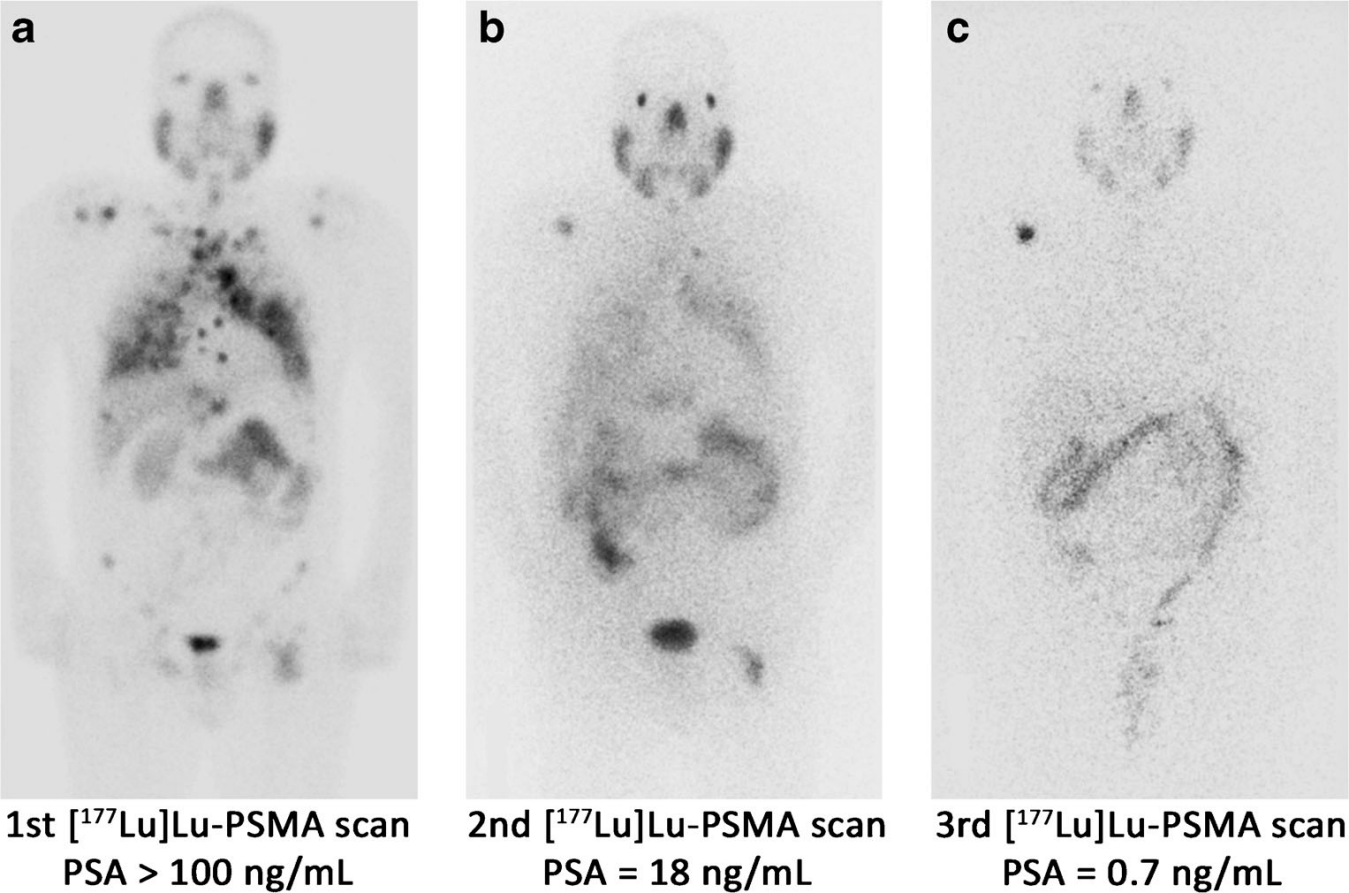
A 56-year-old male with metastatic castration-resistant prostate cancer involving the cervical, mediastinal, and abdominopelvic lymph nodes, multiple bones, and bilateral lungs underwent 3 cycles of Lutetium-177-prostate-specific membrane antigen ([^177^Lu]Lu-PSMA) radionuclide therapy. The sequential post-treatment (PT) planar whole-body [^177^Lu]Lu-PSMA images (a–c) and pre-therapy prostate-specific antigen (PSA) levels indicate a favorable response to treatment
